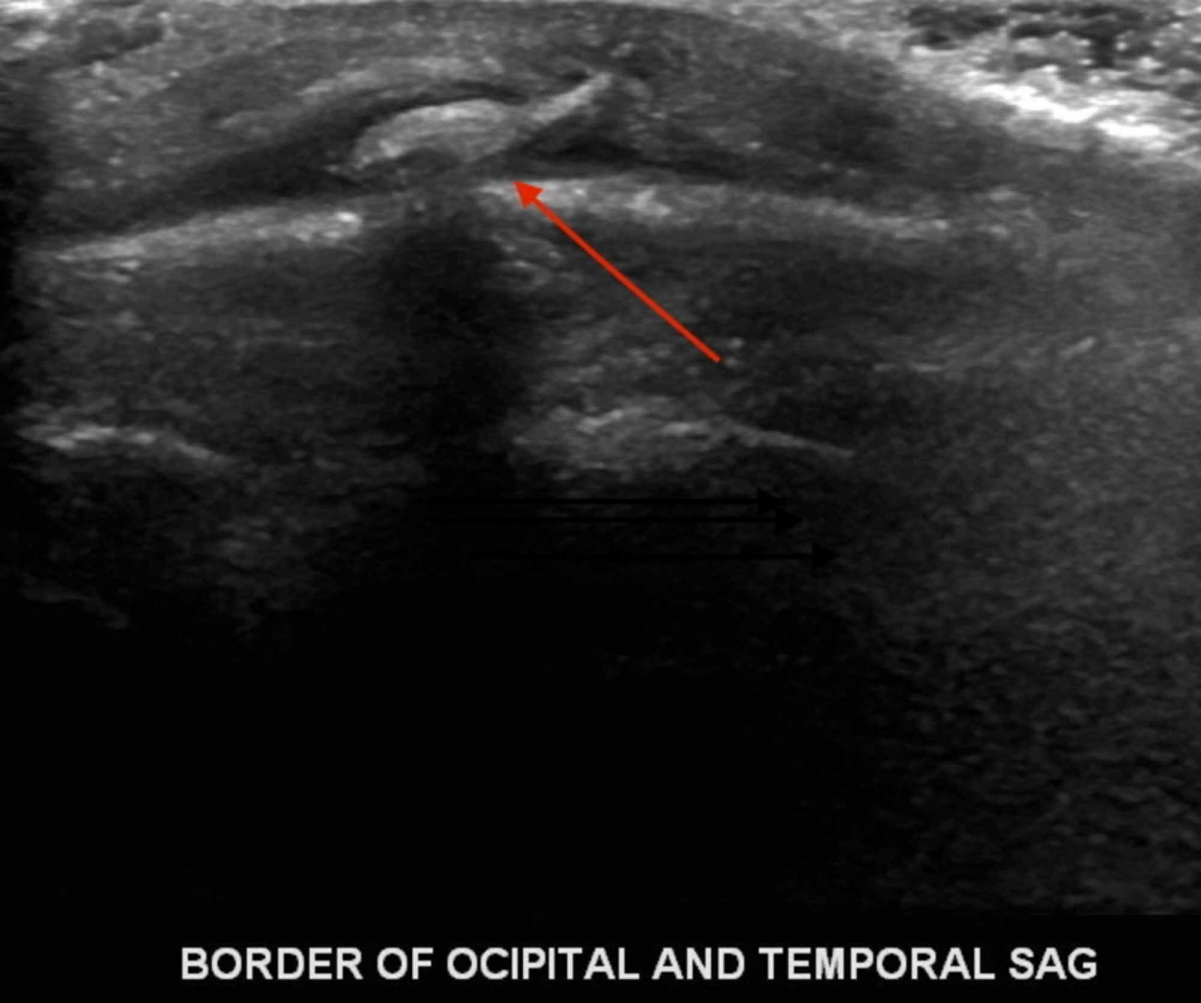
Ultrasound of scalp showing foreign body
